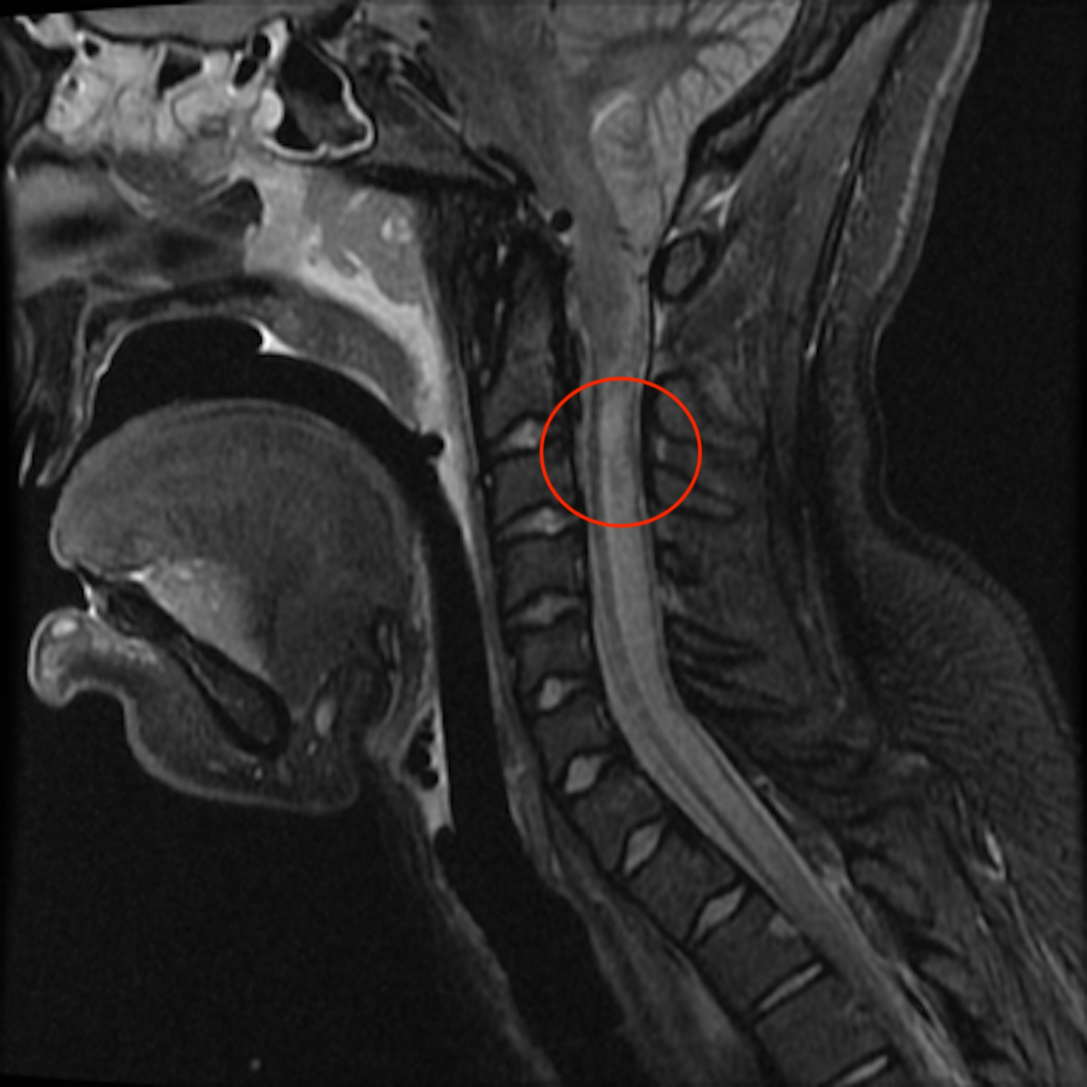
Magnetic resonance imaging (MRI). MRI brain showing Chiari I malformation along with syrinx at C4 and presyrinx down to T2 along with cervical spine venous engorgement and cerebrospinal fluid (CSF) flow obstruction at level of foramen magnum.
